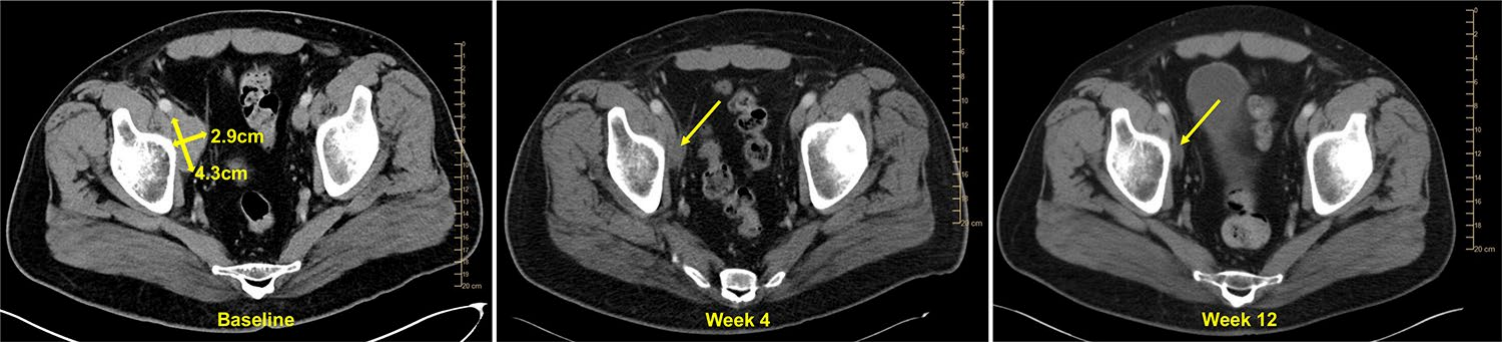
Complete antitumor response in the AMG 232, dabrafenib, trametinib study (Arm 1). Representative images were obtained from patient 6-1011’s CT scans with IV contrast at baseline, week 4, and week 8
